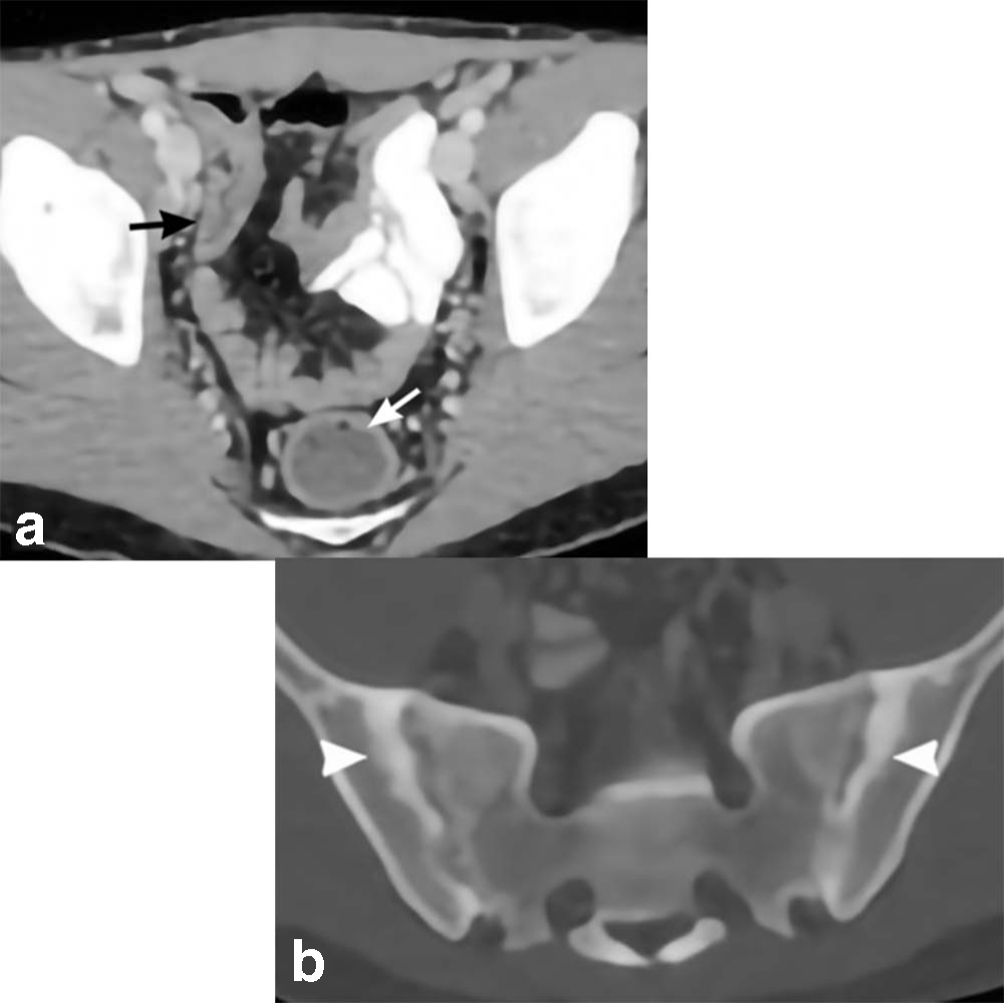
Ulcerative colitis. 24-year-old male presents with abdominal pain and chronic lower back pain. Axial CT in soft tissue windows (a) shows mild diffuse large bowel wall thickening extending from the rectum (white arrow) to the cecum (black arrow). Axial CT in bone windows (b) demonstrates bilateral sacroiliac joint sclerosis and erosions (white arrowheads) consistent with sacroiliitis, a possible extraintestinal manifestation of UC. Biopsy shows chronic moderately active colitis with cryptitis and lymphoid hyperplasia. UC, ulcerative colitis.
